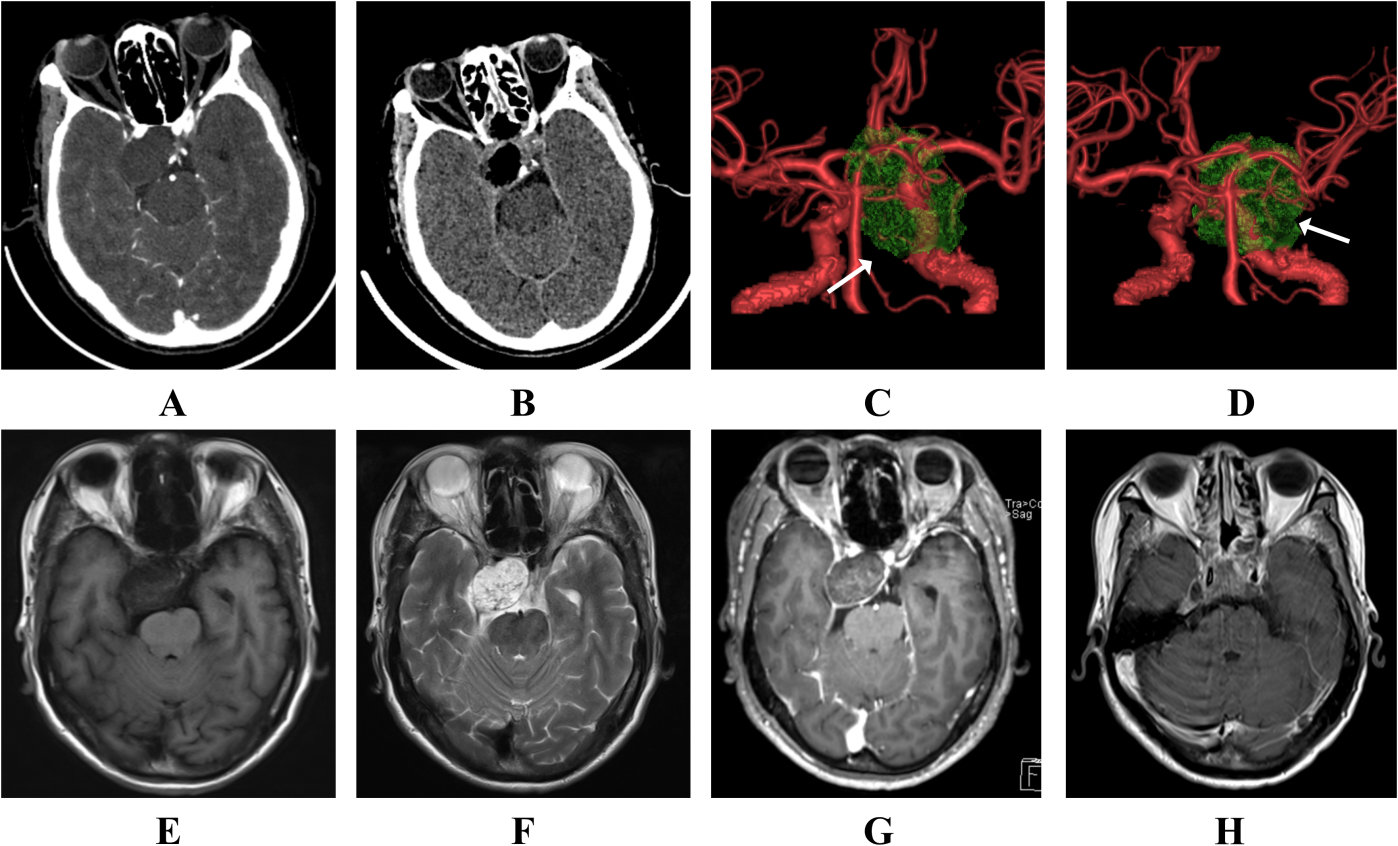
(A) shows the patient’s preoperative cranial CT scan, (B) shows the postoperative cranial CT scan within 24 hours; (C, D) represent the patient’s preoperative cranial CTA images, indicates the tumor invaded the cavernous sinus and encased the right internal carotid artery; (E, F) represents the patient’s preoperative MRI T1-weighted imaging (T1WI) and MRI T2-weighted imaging (T2WI), respectively; (G) shows the preoperative contrast-enhanced MRI of the patient; (H) shows contrast-enhanced MRI at 48 hours postoperatively.
